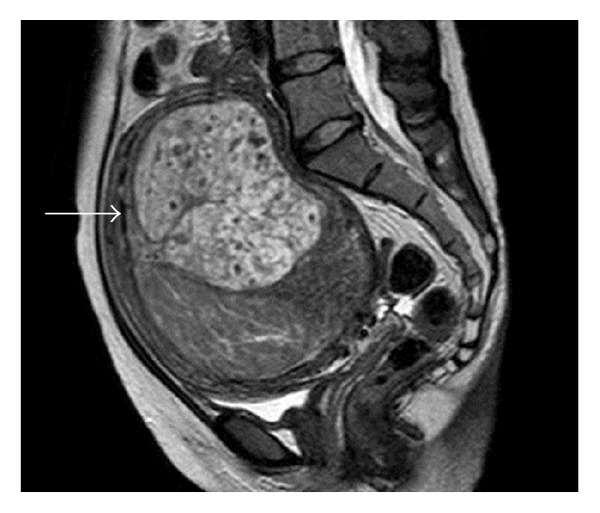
Magnetic resonance imaging of the uterine tumor showing heterogeneous high-signal intensity (arrows) on a T2-weighted image.
